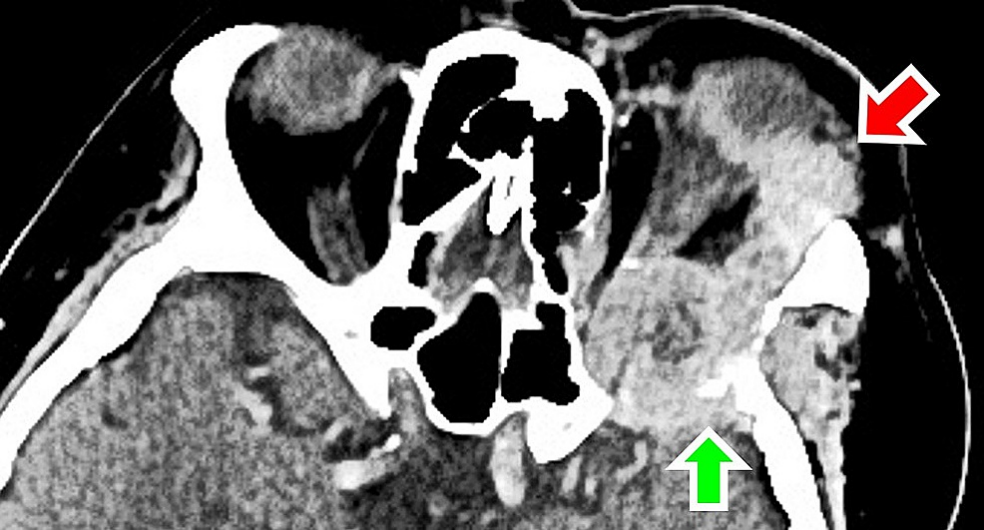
CT orbit showed an orbital mass (red arrow) with lytic destruction of the greater wing of the left sphenoid bone (green arrow).
